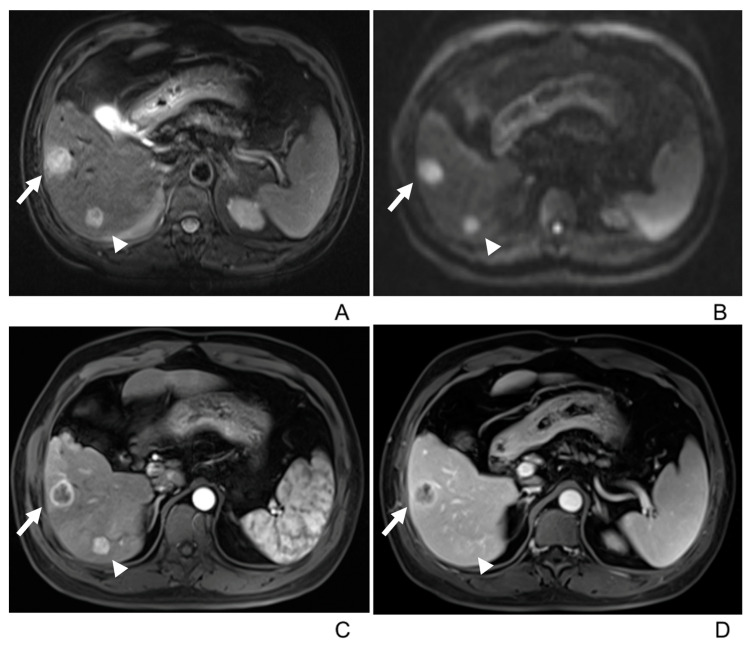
51-year-old male with multiple intrahepatic lymphoepithelioma-like cholangiocarcinomas (>2). (A) T2-weighted MR image displays two well-defined hyperintense tumors in the right lobe. (B) Both tumors show hyperintensity on diffusion-weighted imaging (b = 500 m^2^/s). (C,D) On the arterial and portal venous phase images, the tumor in segment V (arrow) shows peripheral rim arterial-phase enhancement with centripetal progressive enhancement (Type A enhancement pattern), and the tumor in segment VI (arrowhead) shows rapid wash-in and rapid wash-out (Type B enhancement pattern), respectively.
